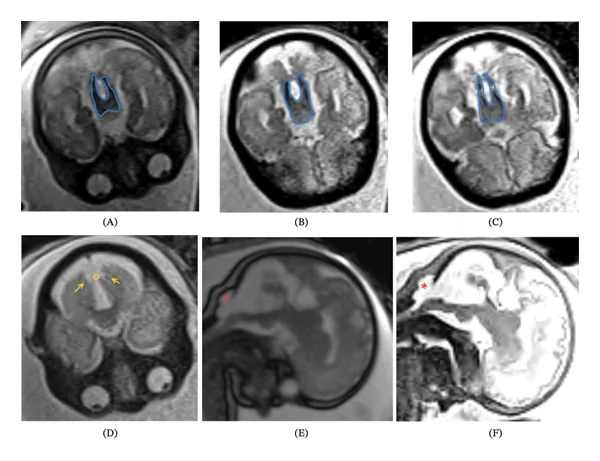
Documented defects in fetal brain MRI: (A, B, C) signs of the “molar tooth” (pathognomonic sign). (D) Agenesis of the vermis of the cerebellum. (E, F) Occipital meningocele.
